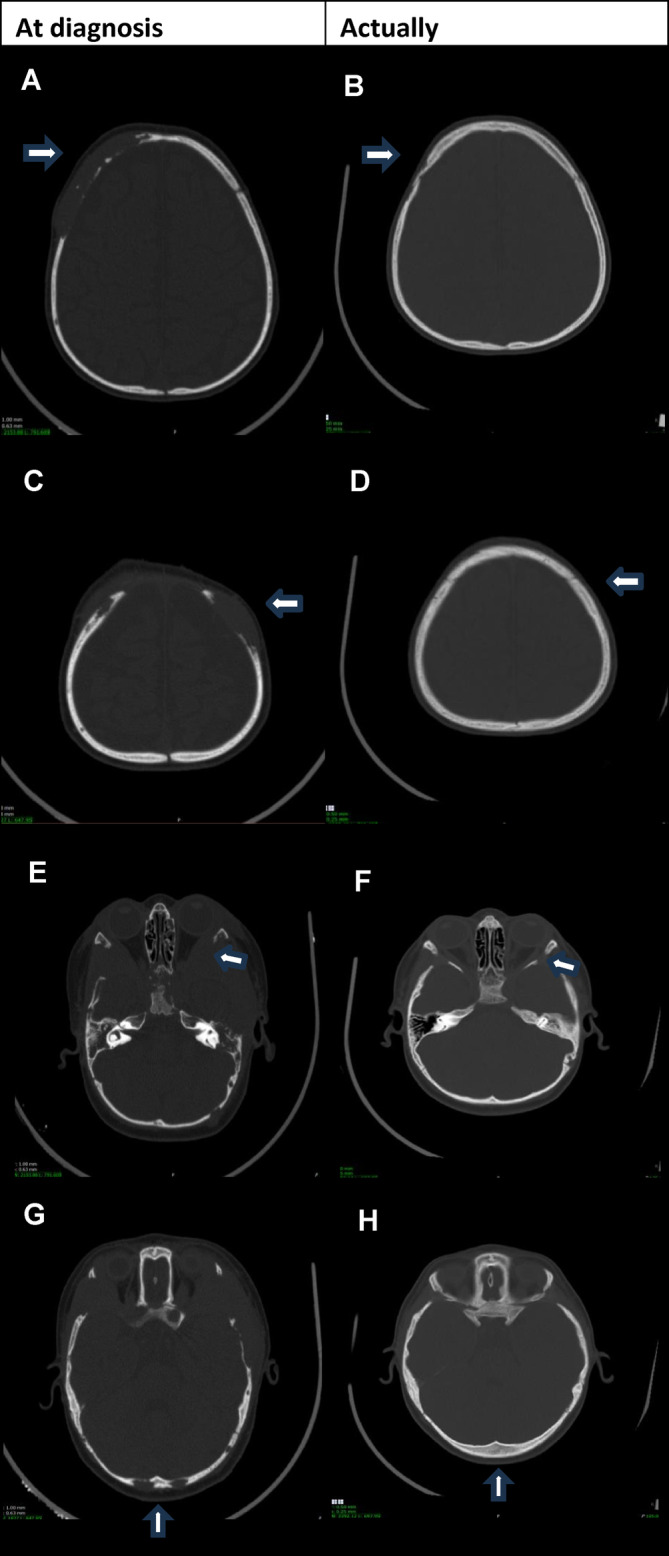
Images from CT scans of the skull that compare the presentation of bone lesions in the skullcap and skull base at the time of diagnosis with the current images (2 years of vemurafenib). (A) Right upper skullcap (extensive osteolytic lesion with expansive soft tissue component). (B) Right upper skullcap (significantly smaller radiolucent bone area). (C) Left upper skullcap (osteolytic lesion with expansive soft tissue component). (D) Left upper skullcap (osteolytic lesion no longer individualizable). (E) Skull base/lateral orbital wall on the left (extensive osteolytic lesion with expansive soft tissue component). (F) Skull base/lateral orbital wall on the left (significantly smaller radiolucent bone area). (G) Bilateral occipital (osteolytic lesions with expansive soft tissue component). (H) Bilateral occipital osteolytic lesions (no longer individualizable).
